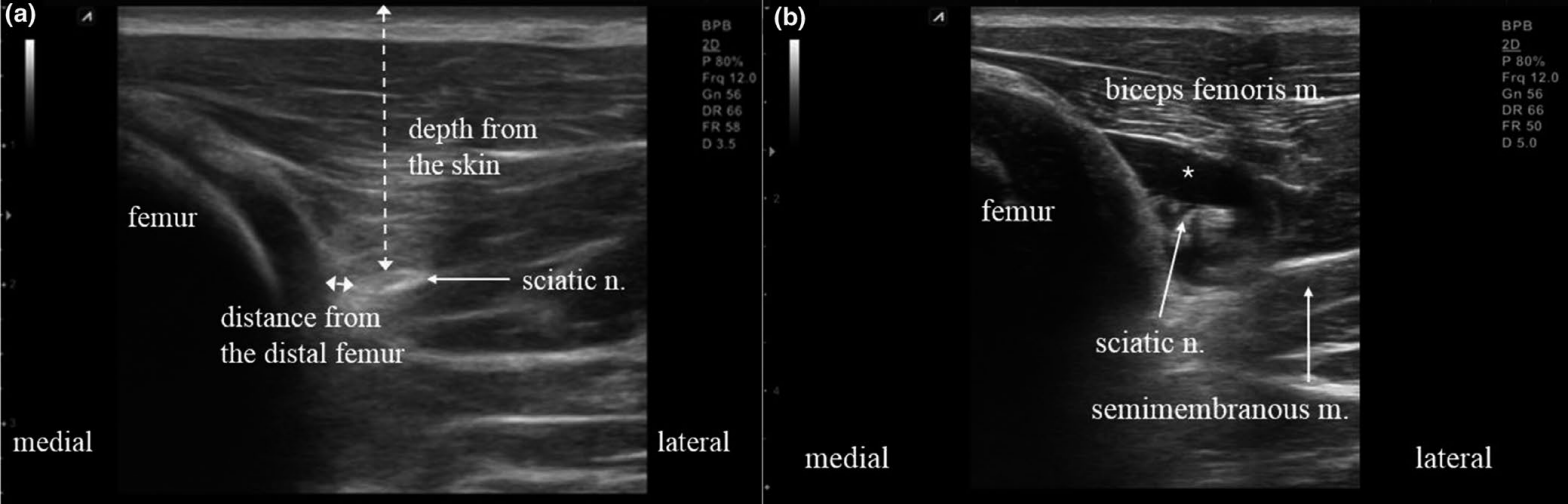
Ultrasound short‐axis images of pre‐ and post‐local anaesthetic injections in the sciatic nerve. (a) pre‐injection sciatic nerve location defined by measuring distances from skin (broken line) and the distal femur (solid line). (b) After injection sciatic nerve which is clearly separated from surrounding tissues (donut sign). *: local anaesthetic
